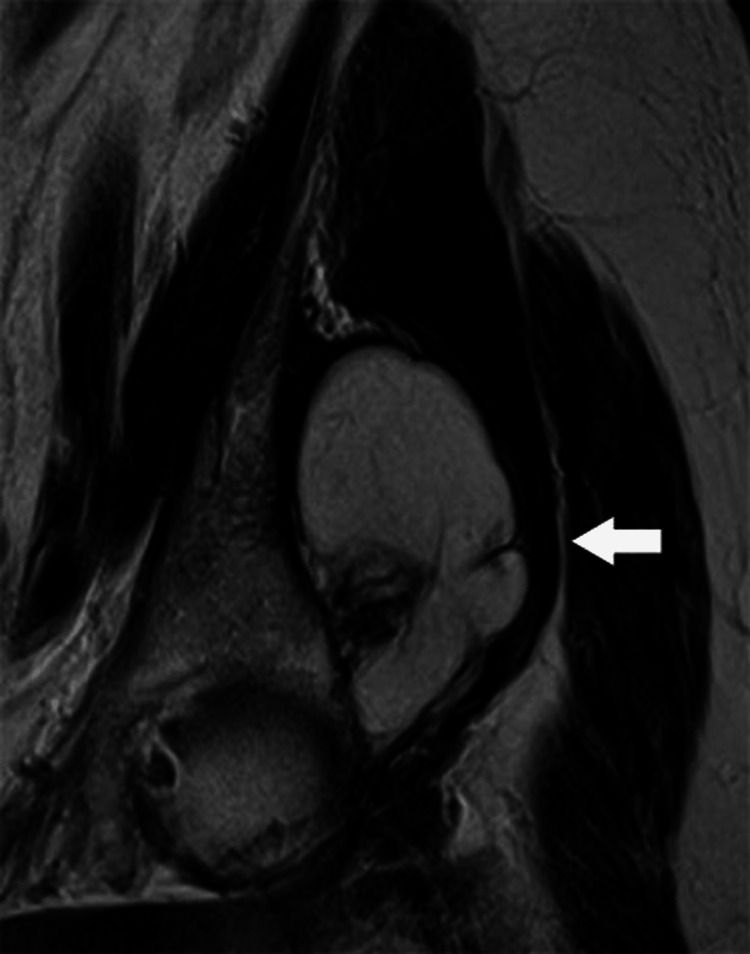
A 60-year-old female with hip pain and inguinal swelling. Coronal T2-weighted MR image shows lipoma (arrow) of arising within the gluteus minimus muscle.
